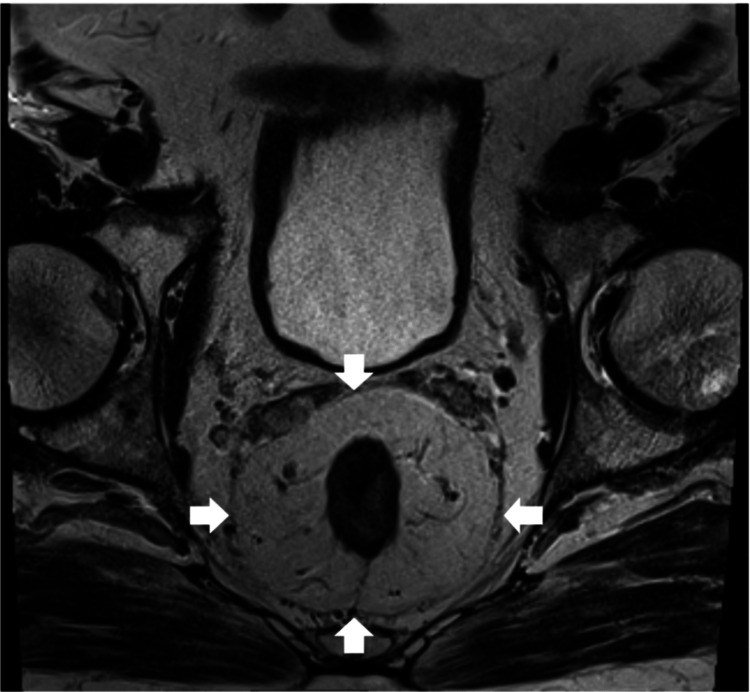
Axial pelvic contrast-enhanced computed tomography demonstrating a circumferential rectal lesion (arrows) without evidence of distant invasion.
